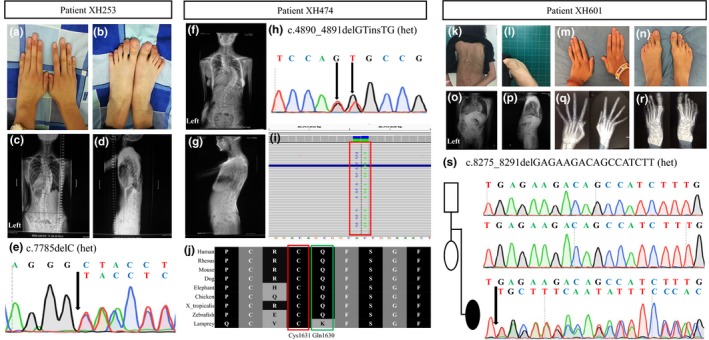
Clinical and genetic manifestations of the research subjects. (a, b) Pictures of Subject XH253 indicated bilateral arachnodactyly of hands and feet. (c, d) The anterior and lateral view of XH253 demonstrated scoliosis on whole spine X‐ray images. (e) Sanger sequencing of XH253 verified a novel heterozygous frameshift variant c.7785delC (p.Tyr2596Thrfs*86) affecting *FBN1*. (f, g) The anterior and lateral view of Subject XH474 demonstrated scoliosis on whole spine X‐ray images. (h) Sanger sequencing verified monoallelic missense mutations c.4890_4891delGTinsTG (p.Gln1630_Cys1631delinsHisGly) affecting *FBN1*. (i) Integrative Genomics Viewer (IGV) displays varying level of alignment reads detail depending on the zoom level and uses red box and transparency to highlight monoallelic missense variants in the exome data from XH474. (j) Conservation analysis of amino acid residues of *FBN1* among vertebrates. Lines indicate homologous amino acid sequences in selected vertebrates. Note the strong conservation of Cys1631 among vertebrates highlighted in red and Gln1630 highlighted in green, respectively. (k, l, m, n) Photographic images of Subject XH601 who presented with scoliosis, short stature and subcutaneous fat reduction and arachnodactyly anomalies of hands and feet. (o, p) The anterior and lateral view demonstrated severe scoliosis on whole spine X‐ray images. (q, r) Hand and foot radiographs showed bilateral metacarpophalangeal dislocation, interosseous atrophy, claw hands, and dolichostenomelia. (s) Sanger sequencing of XH601 parent–offspring trio verified a novel de novo heterozygous frameshift mutation c.8275_8291delGAGAAGACAGCCATCTT (p.Glu2759Cysfs*9) affecting *FBN1*.
